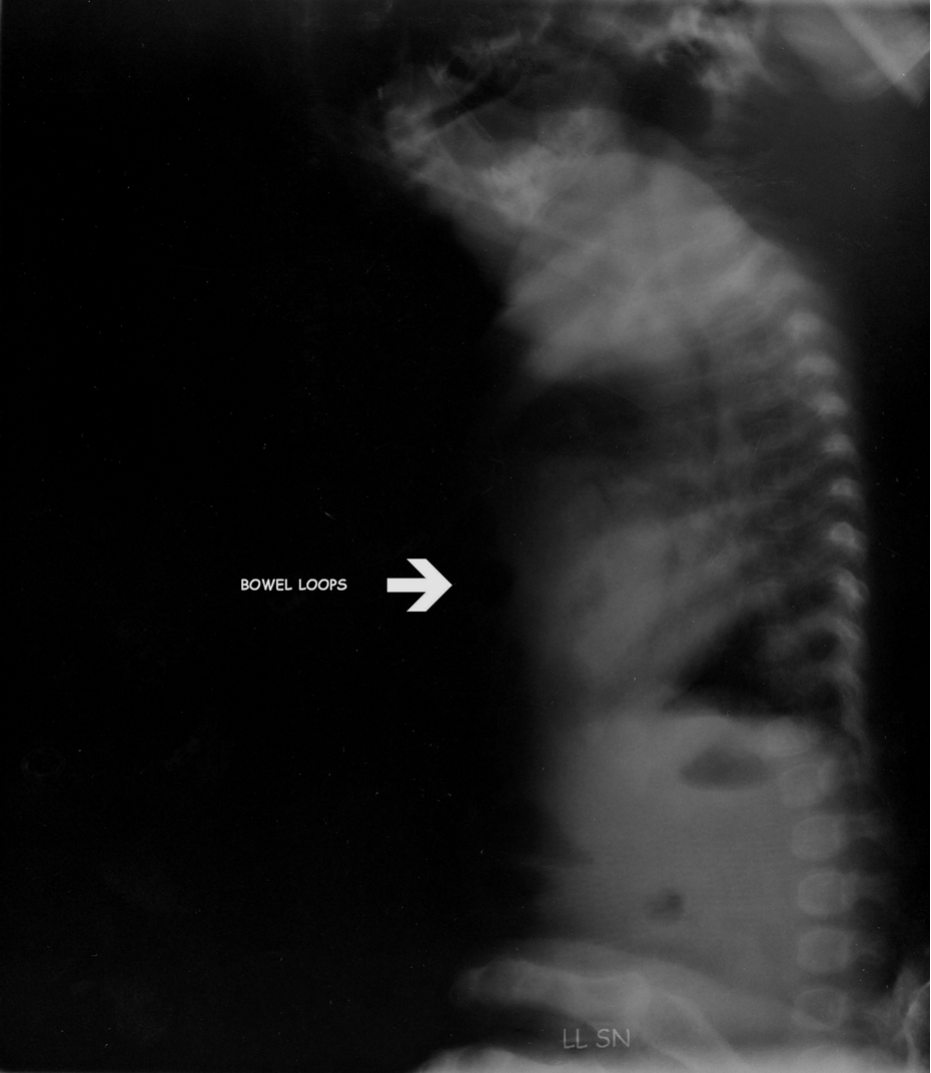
Lateral chest X-ray. Bowel loops compressing the right ventricle of the heart (white arrow).
